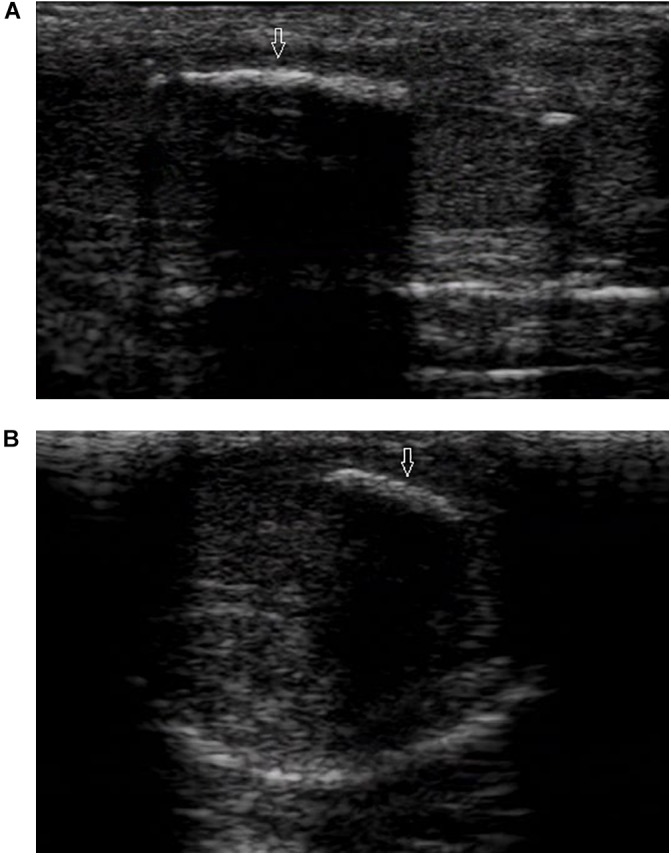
A large calcified lesion in the tunica albuginea of the flaccid penis. (A) Longitudinal sonogram, dorsal access. Large calcified plaques (arrow) in the dorsal side with strong shadow caused dorsal curvature of the penis. (B) Axial sonogram, dorsal access. Plaque located in the tunica albuginea of left corpora cavernosa.
